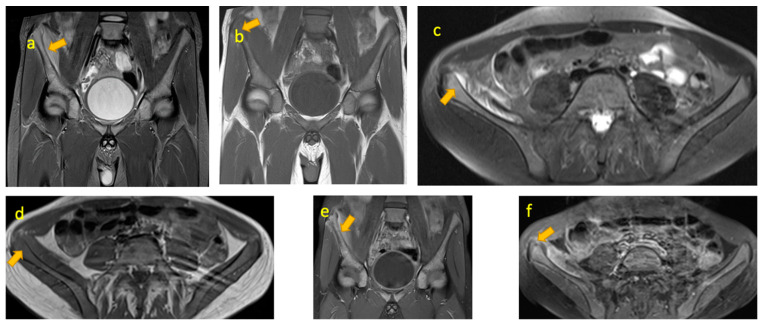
(a–f) Coronal STIR, coronal T1W, axial T2W FS, and axial T1W MRI images of a 12-year-old boy with acute osteomyelitis of the right iliac crest apophysis. MRI images depict bone marrow oedema signal in the right iliac crest adjacent to the apophysis, a small subperiosteal abscess on the medial side, and severe oedema of the right iliacus muscle.
